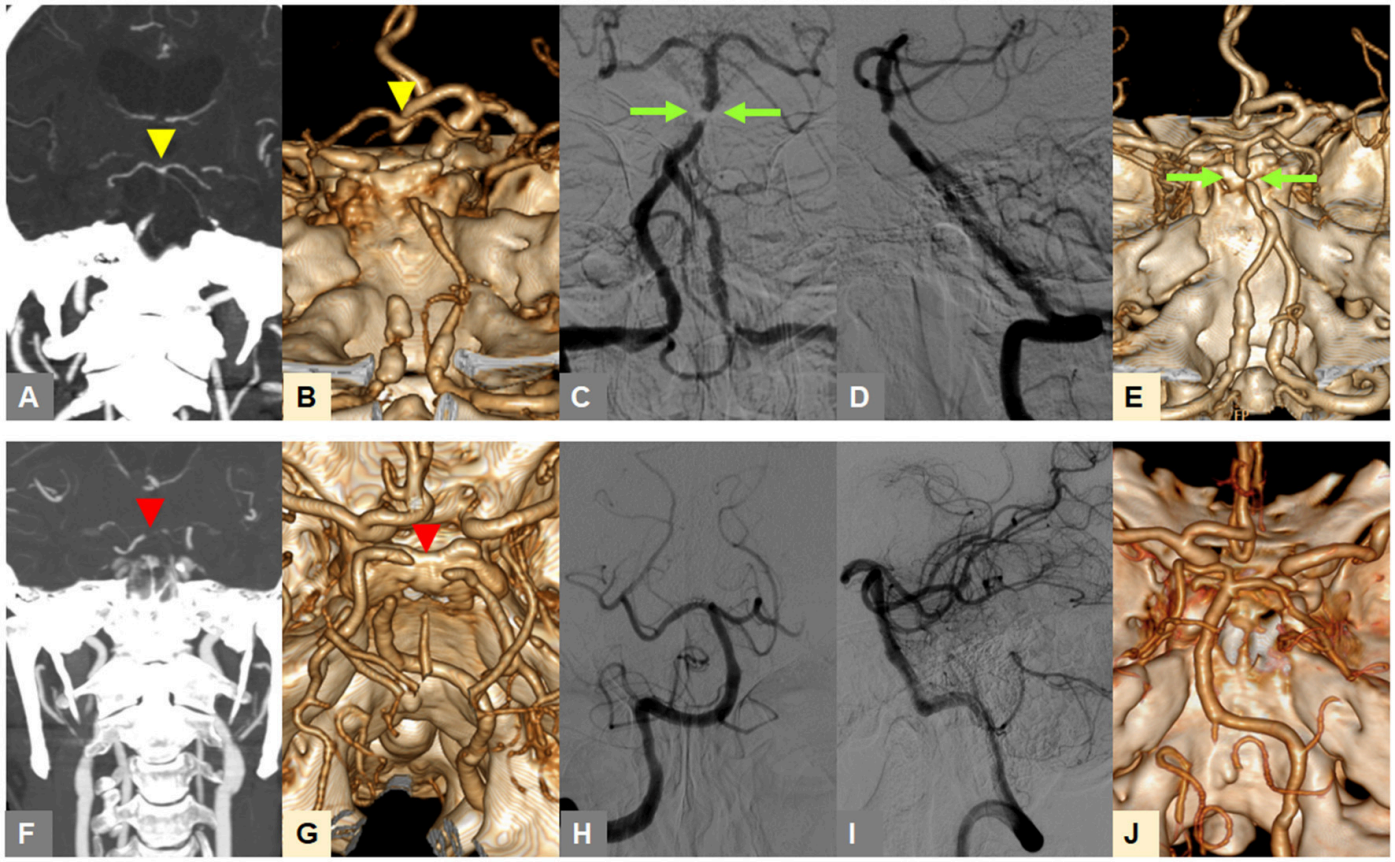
Representative cases for classification of occlusion types on baseline CTA and angiography-revealed fixed focal stenosis. (A,B) A truncal-type occlusion of the basilar artery, with clearly visible bifurcation point at the top of the basilar artery (yellow arrowheads) is observed on baseline CTA. (C,D) Digital subtraction angiography after reperfusion treatment reveals >70% residual stenosis (green arrow). (E) Post-procedure CTA shows underlying atherosclerotic lesion (green arrow). (F,G) A branch-site occlusion of the basilar artery with involvement of the top of the basilar portion and P1 segments (red arrowheads) is observed. (H,I) Complete recanalization after primary thrombectomy is seen, suggestive of embolic etiology. (J) Post-procedure CTA also shows complete recanalization. CTA, computed tomographic angiography.
